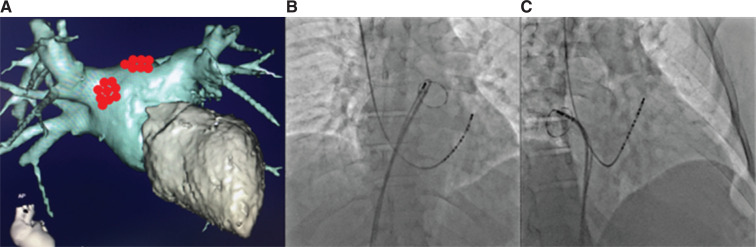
A: Three-dimensional reconstruction of LA and left ventricular computed tomography angiography in the anteroposterior view; red dots show the regions of additional RF lesions aiming at the right superior and left superior GPs. B: Chest X-ray in the anteroposterior view, demonstrating the position of the mapping catheter during RF ablation in the region of the left superoposterior GP. A Lasso catheter in the left superior PV ostium and a decapolar catheter in the coronary sinus (CS) are also shown. C: Chest X-ray in the right oblique view, demonstrating the position of the mapping catheter during RF ablation in the region of the right anterosuperior GP. A Lasso catheter in the right superior PV ostium and a decapolar catheter in the CS are also shown.
